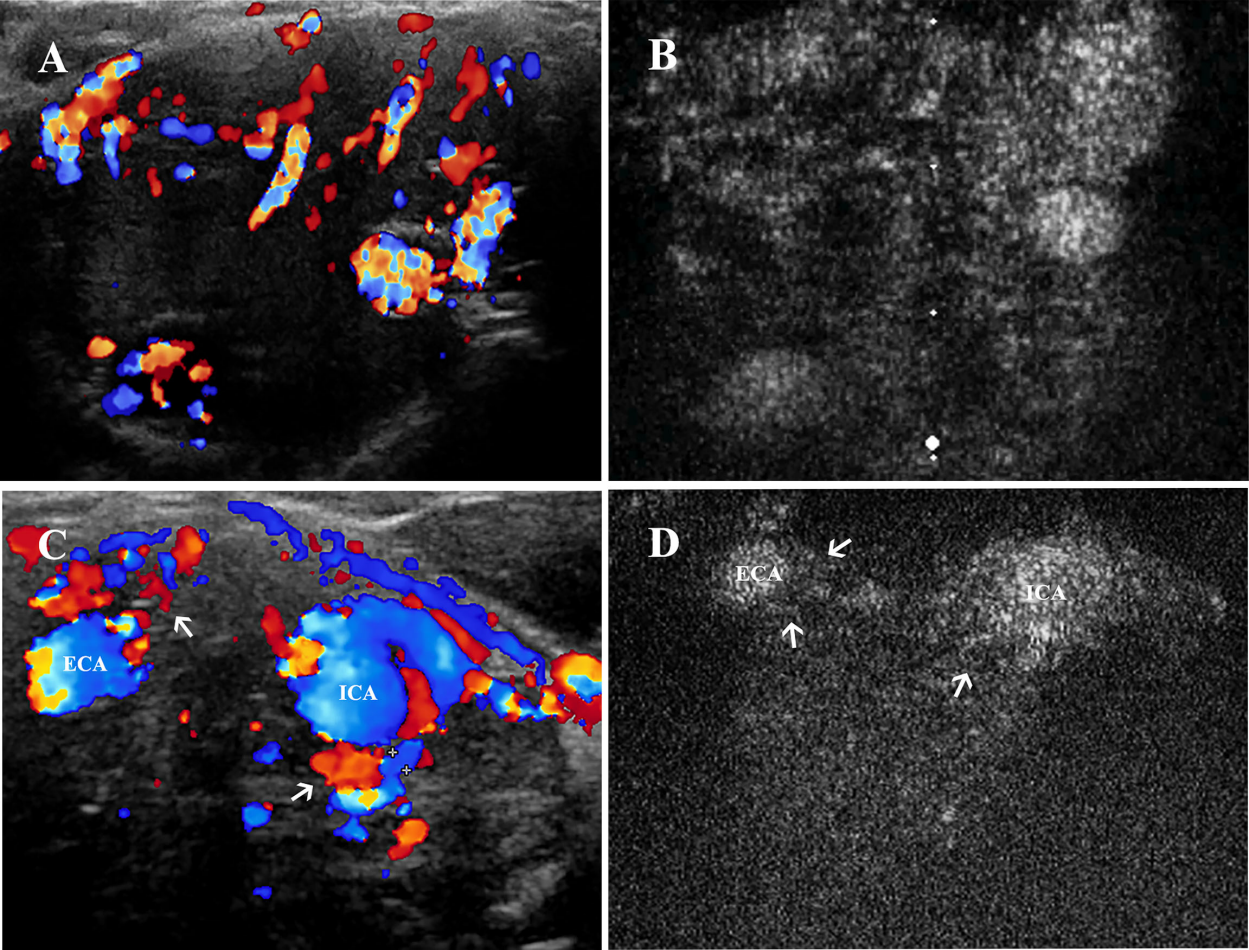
Vascularity and feeding vessels of CBT lesions. (A) CDU showed a hypervascularity and hypoechoic mass located at carotid bifurcation, and (B) robust signal intensity could be seen 1–2 s after the enhancement of carotid arteries on CEUS. (C) Feeding vessels (thin arrows) of another CBT lesion could be observed deriving from both ECA and ICA on CDU and (D) on CEUS. CBT, carotid body tumor; CEUS, contrast-enhanced ultrasonography; CDU, color Doppler ultrasound; ICA, internal carotid artery; ECA, external carotid artery.
